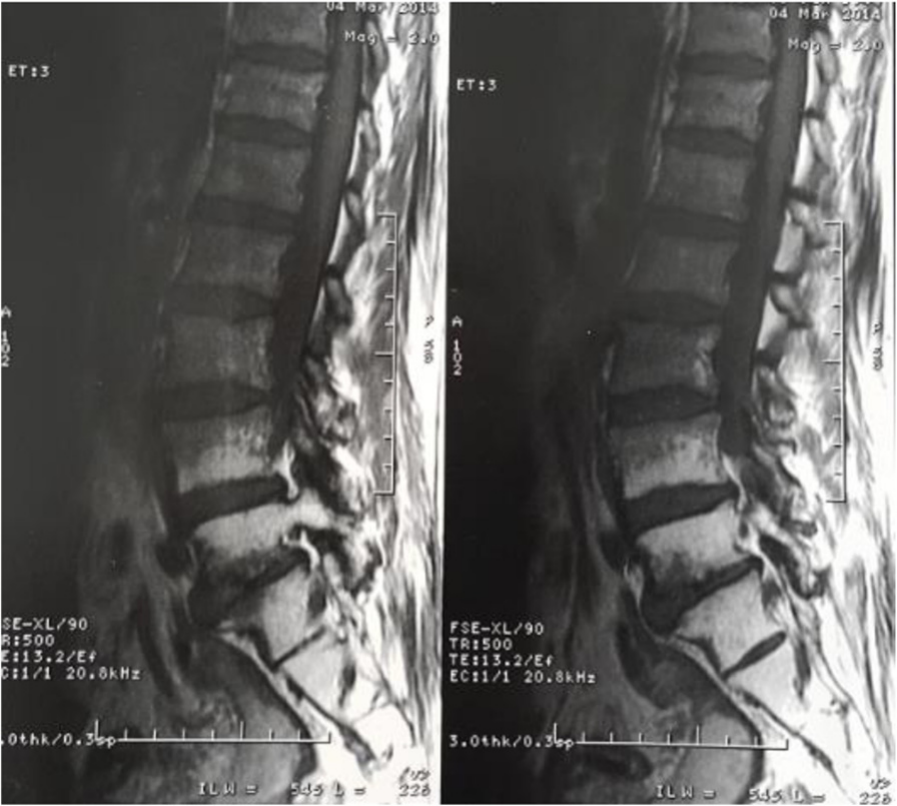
Spinal cord MRI T1-weighted sequence
